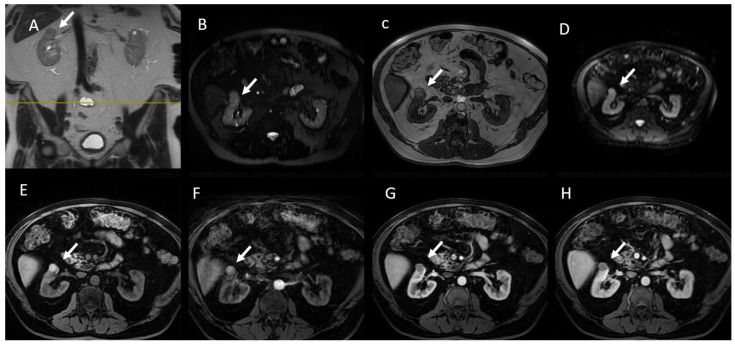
MRI assessment of small renal lesion: hemorrhagic cysts (arrows). The lesion is hypointense on T2W sequences (A,B) and hyperintense in T1W sequences without contrast agents (C,E), with restricted diffusion (D) and without contrast enhancement during contrast study ((F): arterial phase; (G): corticomedullary phase and (H): portal phase).
